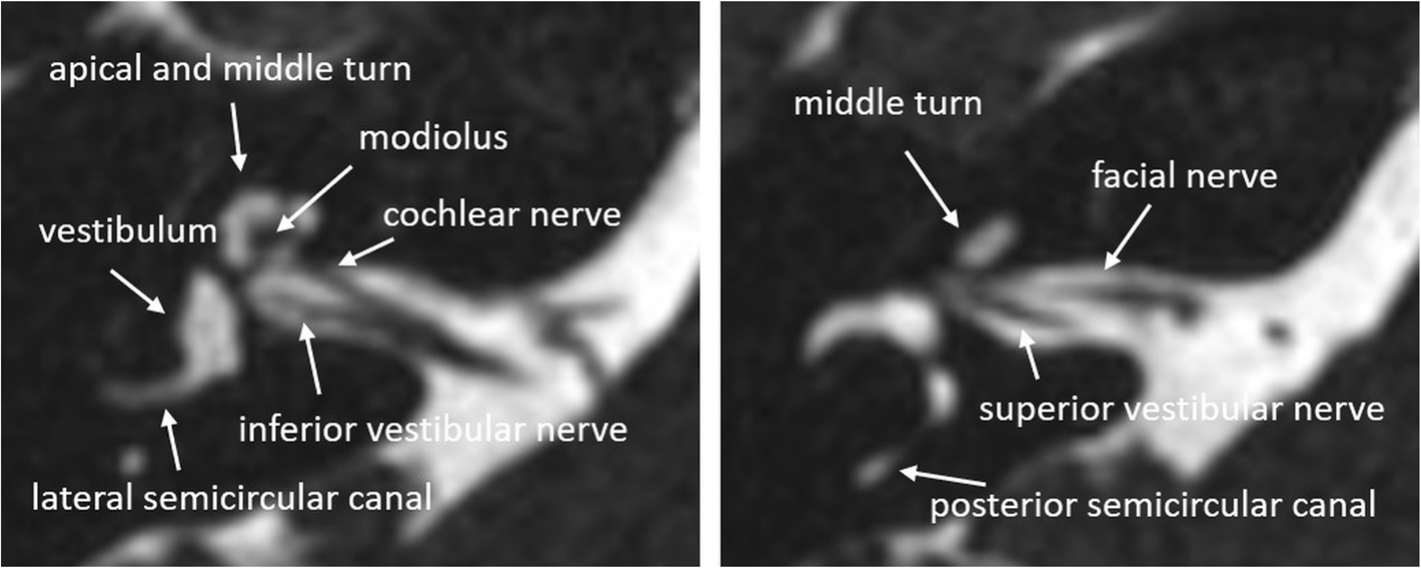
MRI 3D-CISS axial images of normal cochlear anatomy in a 40-year-old woman
